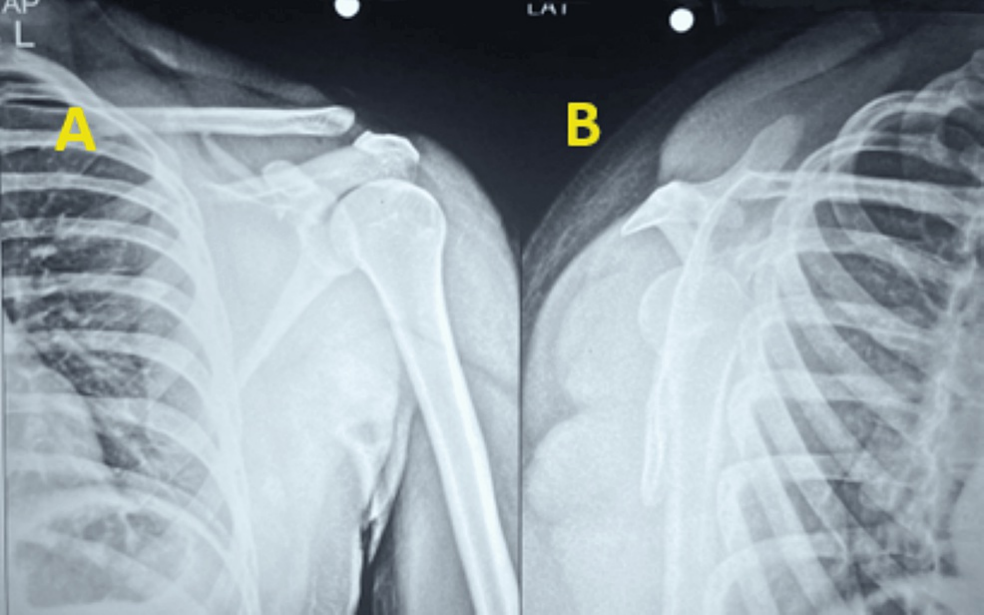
Plain radiographs showing anteroposterior view and lateral scapular view A) Anteroposterior view; B) Lateral scapular view
